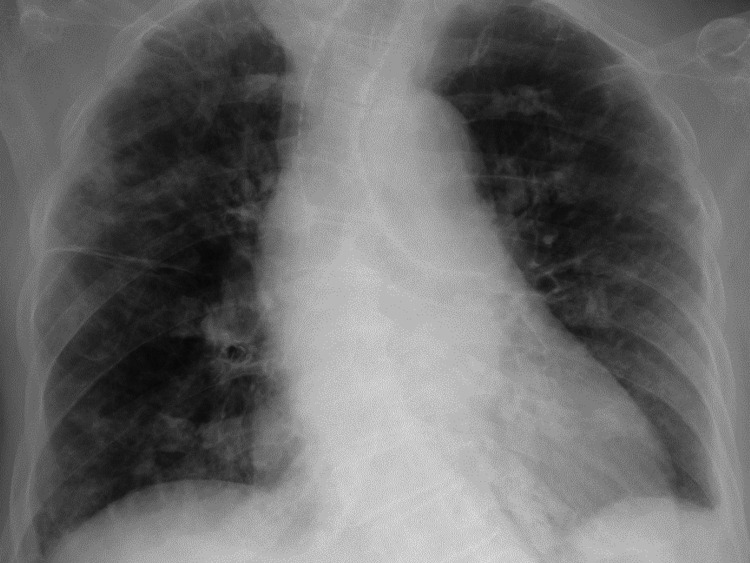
Initial chest radiograph showing bilateral pulmonary infiltrates
